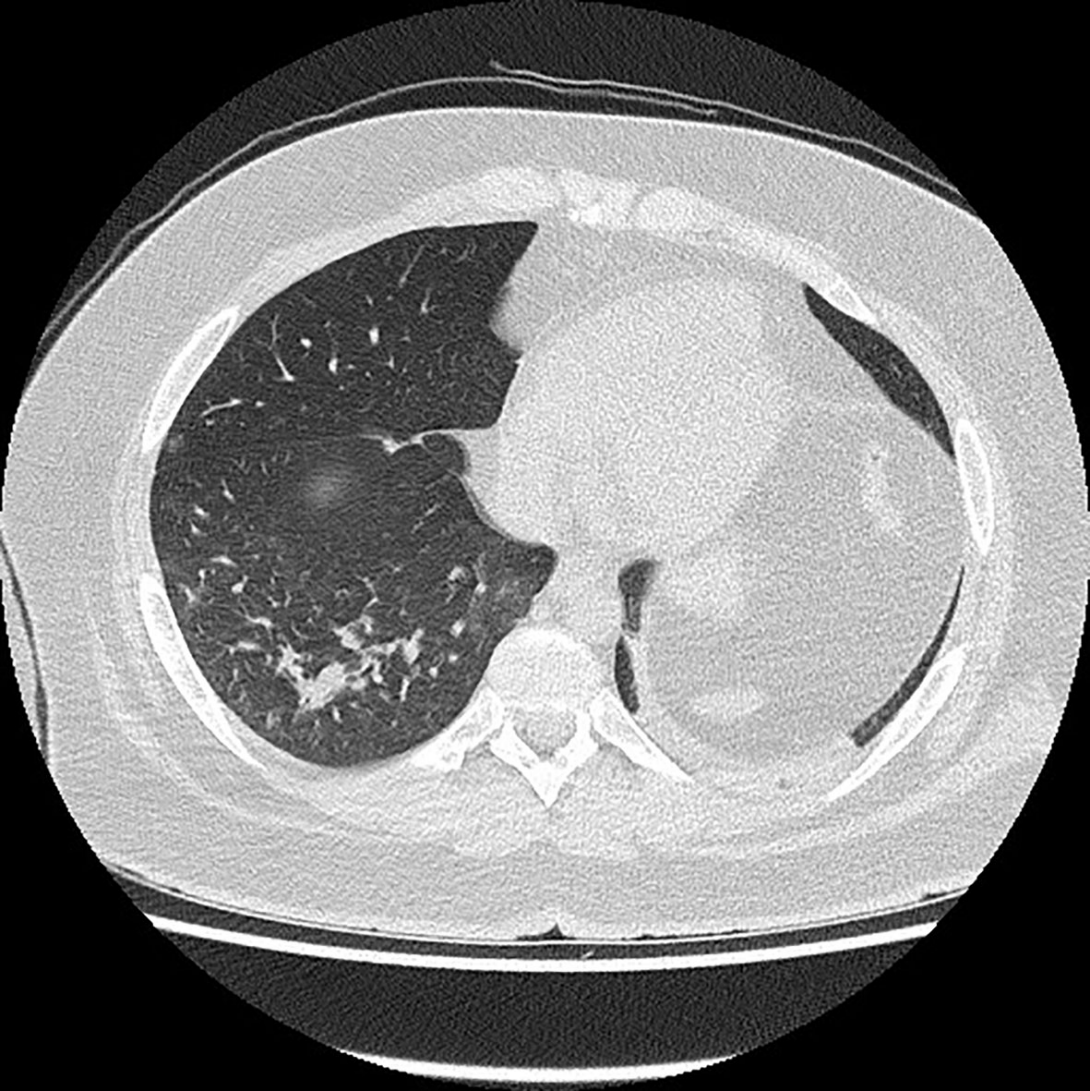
Chest computed tomography scan.
